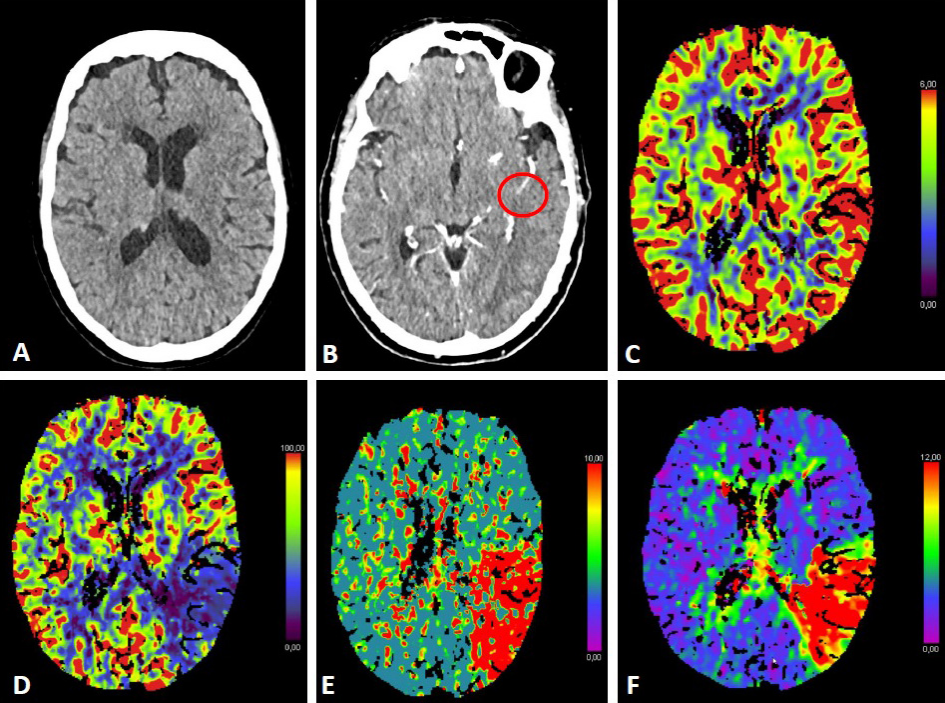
CT-Perfusion. NCCT ASPECTS 10 (A). CT-A with M2-occlusion (B).
